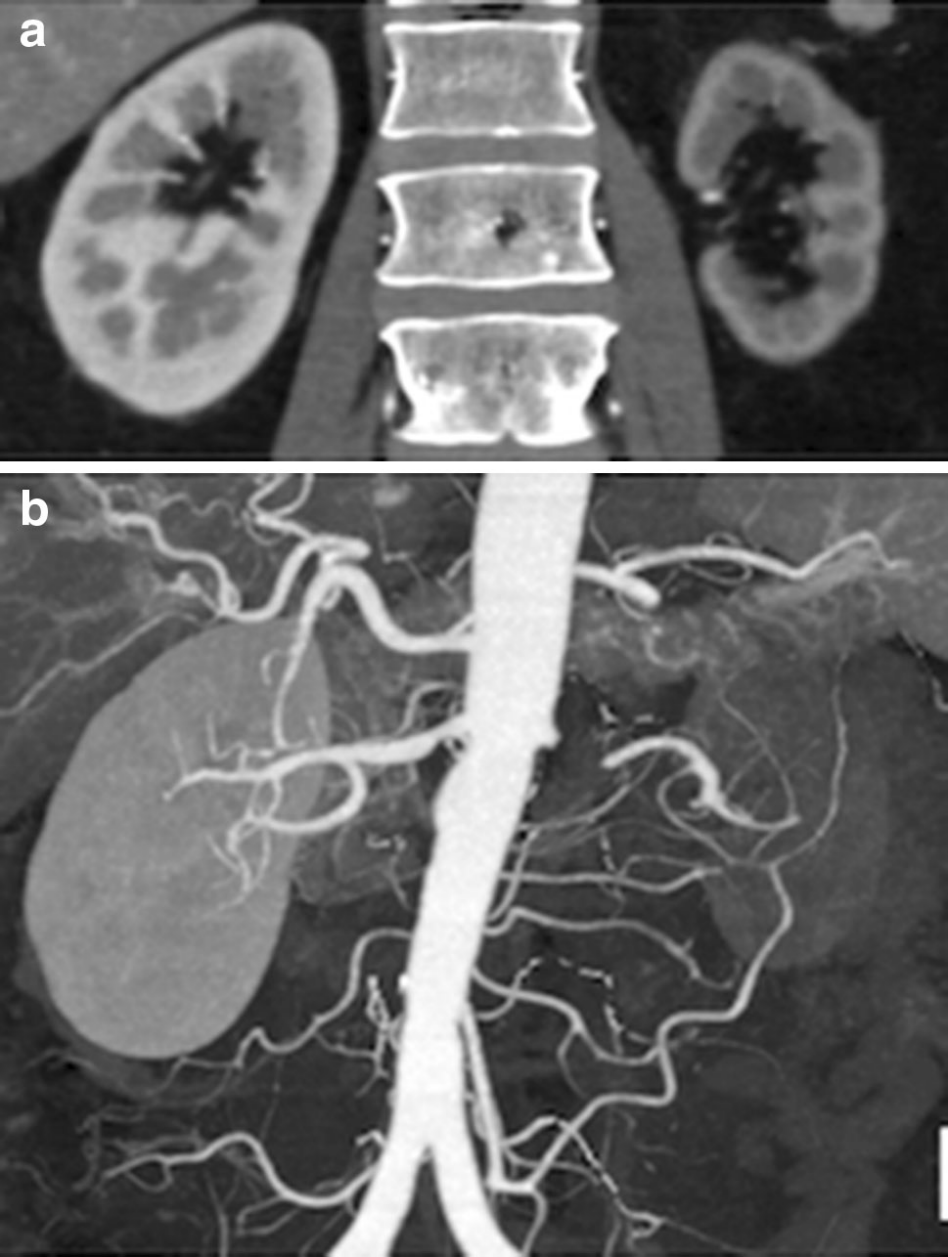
Multidetector-row computed tomography images of the kidneys and vessels demonstrating occlusion of left renal artery, atrophy of left kidney, and mild stenosis of right renal artery. a Coronal plane view. b Three-dimensional view. Note that vessels collateral to the left renal artery around the left kidney were rich
